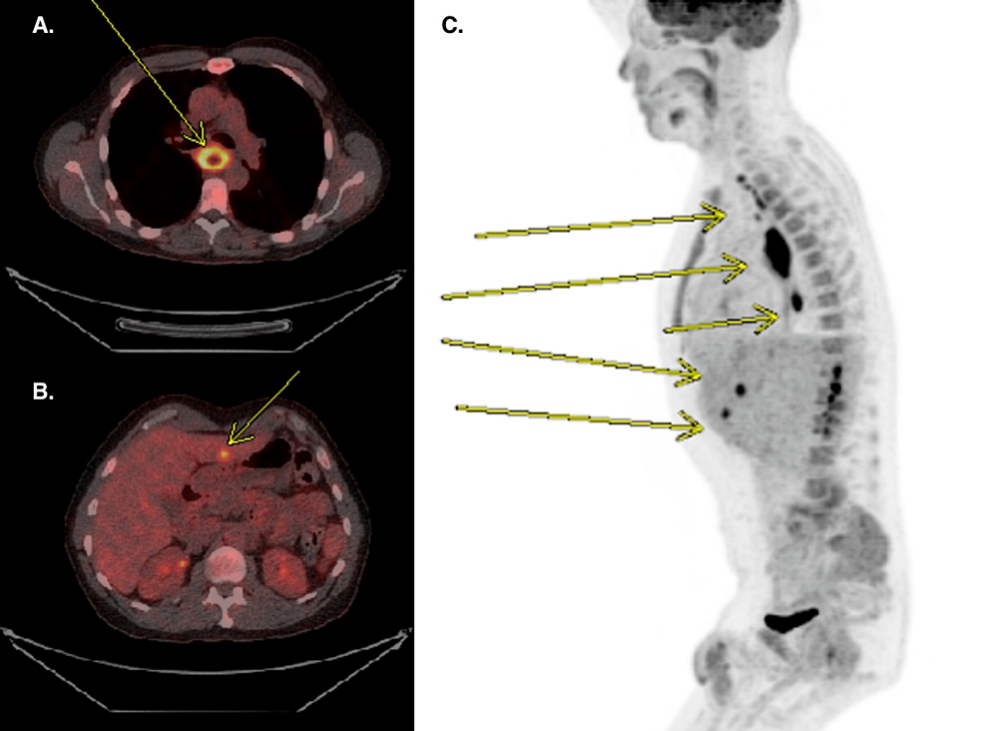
PET-CT A: circumferential mid-esophageal mass approximating 3 cm in diameter and 6 cm in length, SUV 8.7 units B: Liver metastasis C: Abnormal metabolically active lesions consistent with disease in the esophagus, subcarinal and paraesophageal lymph nodes
